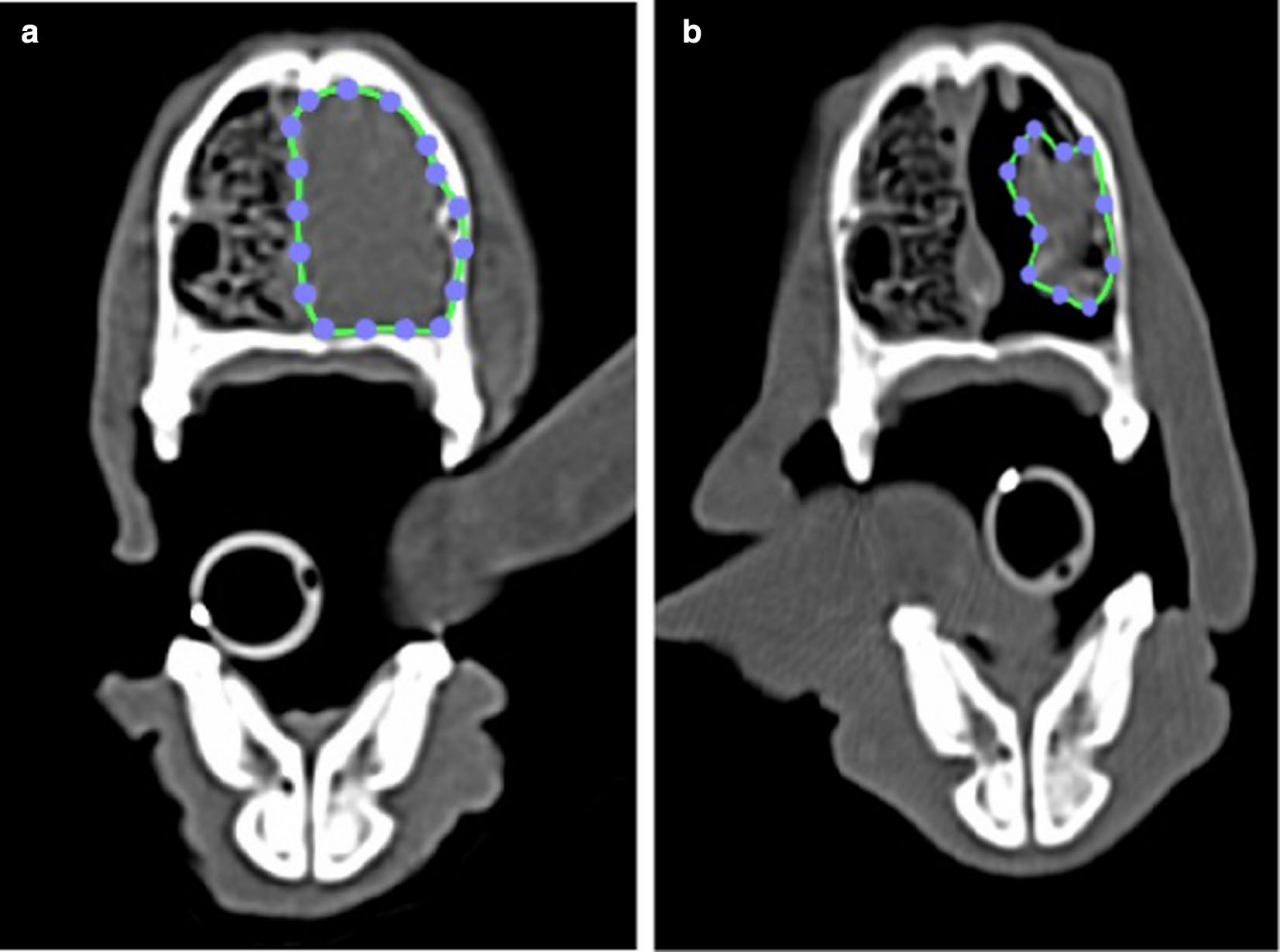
(Patient #1) a pre radiotherapy CT scan, b post radiotherapy CT scan (1 month post radiation therapy)
